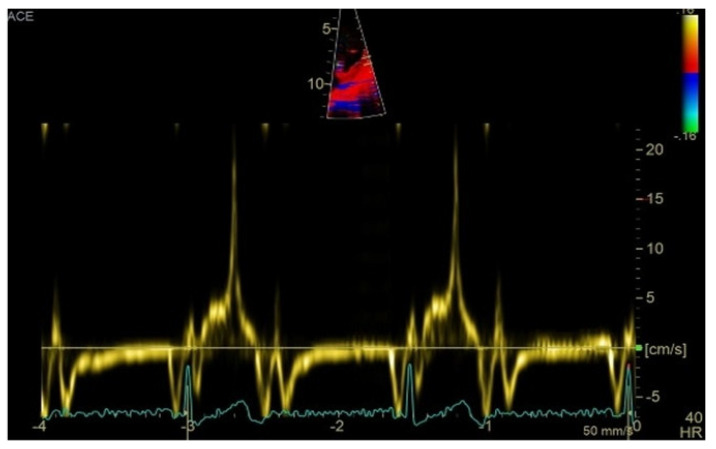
High-velocity spike (21 cm/s) between mid-systole to late-systole using tissue Doppler imaging on the lateral mitral annulus. Known as the Pickelhaube sign, this sharp annular spike resembles the spike that adorns the Pickelhaube helmet, historically worn by the German military. It has been proposed as a marker of arrhythmogenic mitral valve prolapse.
